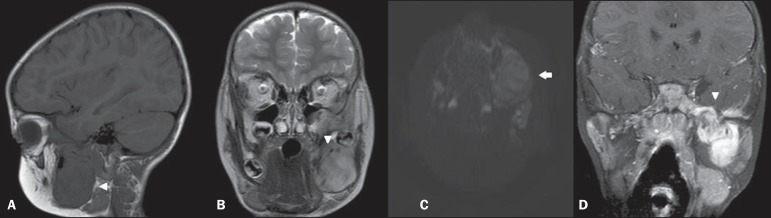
A: Sagittal, T1-weighted image showing lesion with hyposignal affecting
